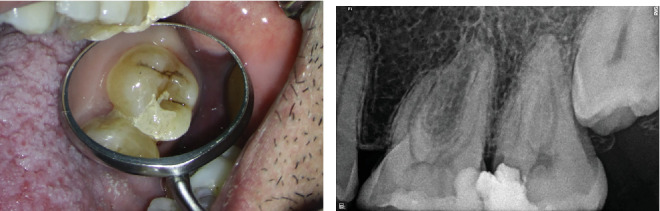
(a) Intraoral photographs of Tooth #15 prior to treatment. (b) Periapical radiograph captured before initiating root canal therapy.
